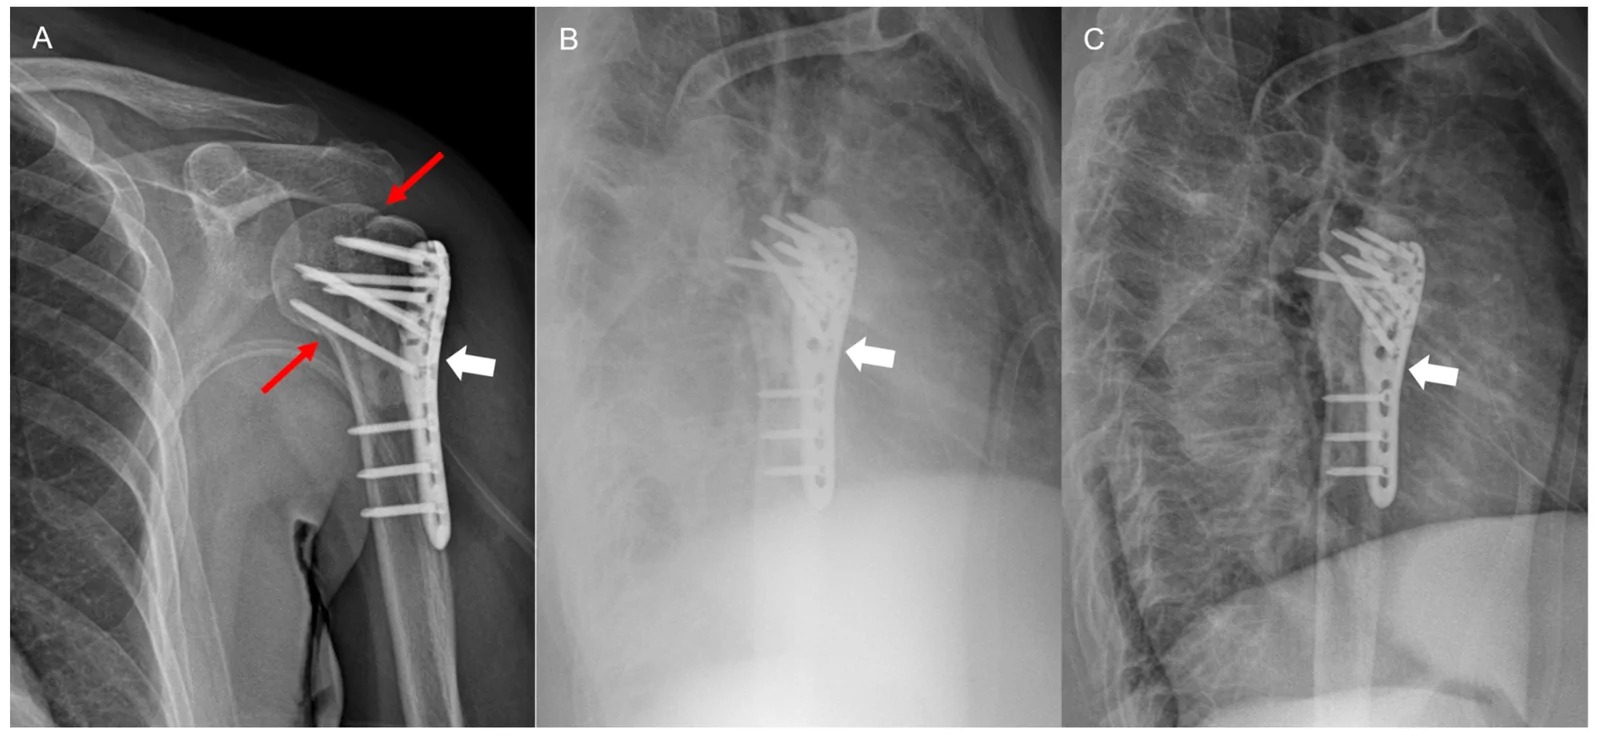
Shoulder radiographs of a 46-year-old male patient after humeral head fracture fixation. (A) Anteroposterior shoulder projection demonstrated the humeral head fracture (red arrow) and the metallic implant (white arrow). Compared with the free-breathing image (B), the deep-inspiration breath-hold image (C) showed greater lung expansion and a lower diaphragmatic position, resulting in a more radiolucent thoracic background and reduced superimposition of thoracic soft tissues over the proximal humerus. The cortical margins of the proximal humerus and the fixation metallic implant were more clearly delineated (white arrows), with improved image contrast and less motion-related blurring.
